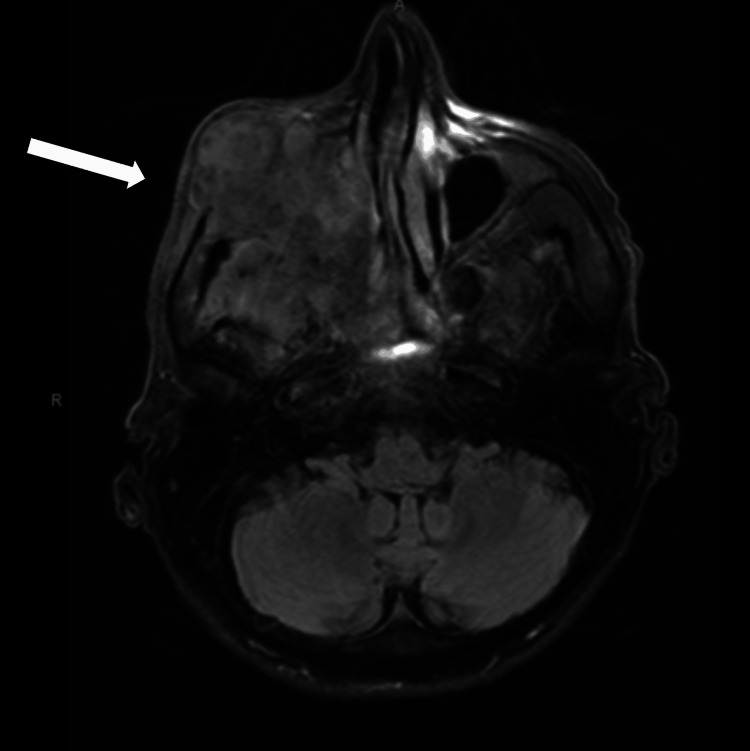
MRI of the brain with and without contrast. MRI brain revealed a large 7.1x5.7x5.2 cm mass centered in the right maxillary sinus with invasion of the nasal cavity, ethmoid and sphenoid sinuses, orbital apex, cavernous sinus, masticator space, multiple skull base foramen, and intracranial extension.
